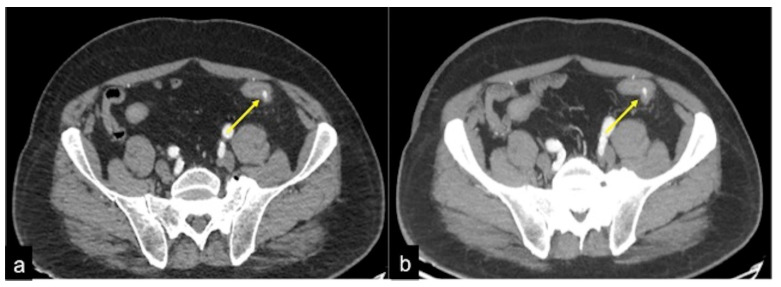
CTA arterial phase (a) and relative MIP reconstruction (b) of a haemorrhagic spot (a,b, arrow) in the descending colon.
